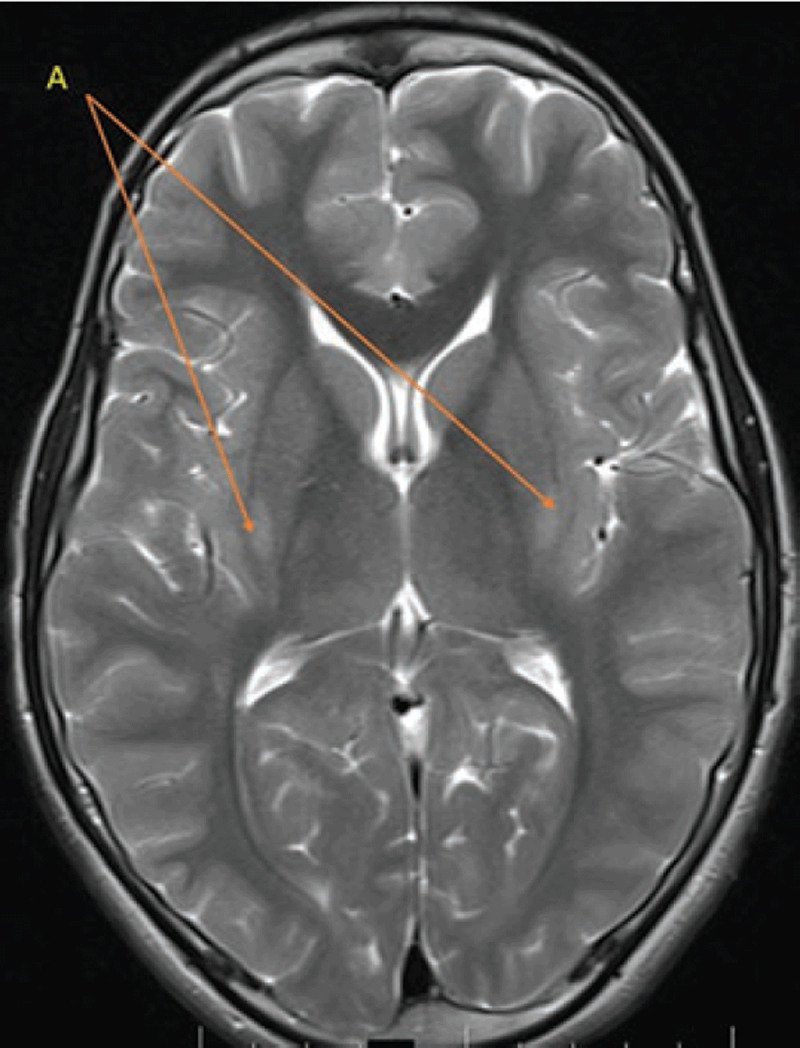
Selective MRI Image is Displayed with Salient Features. (A) The axial T2 fluid-attenuated inversion recovery sequence showing hyperintensity in the putamen bilaterally suggesting anoxic injury.
